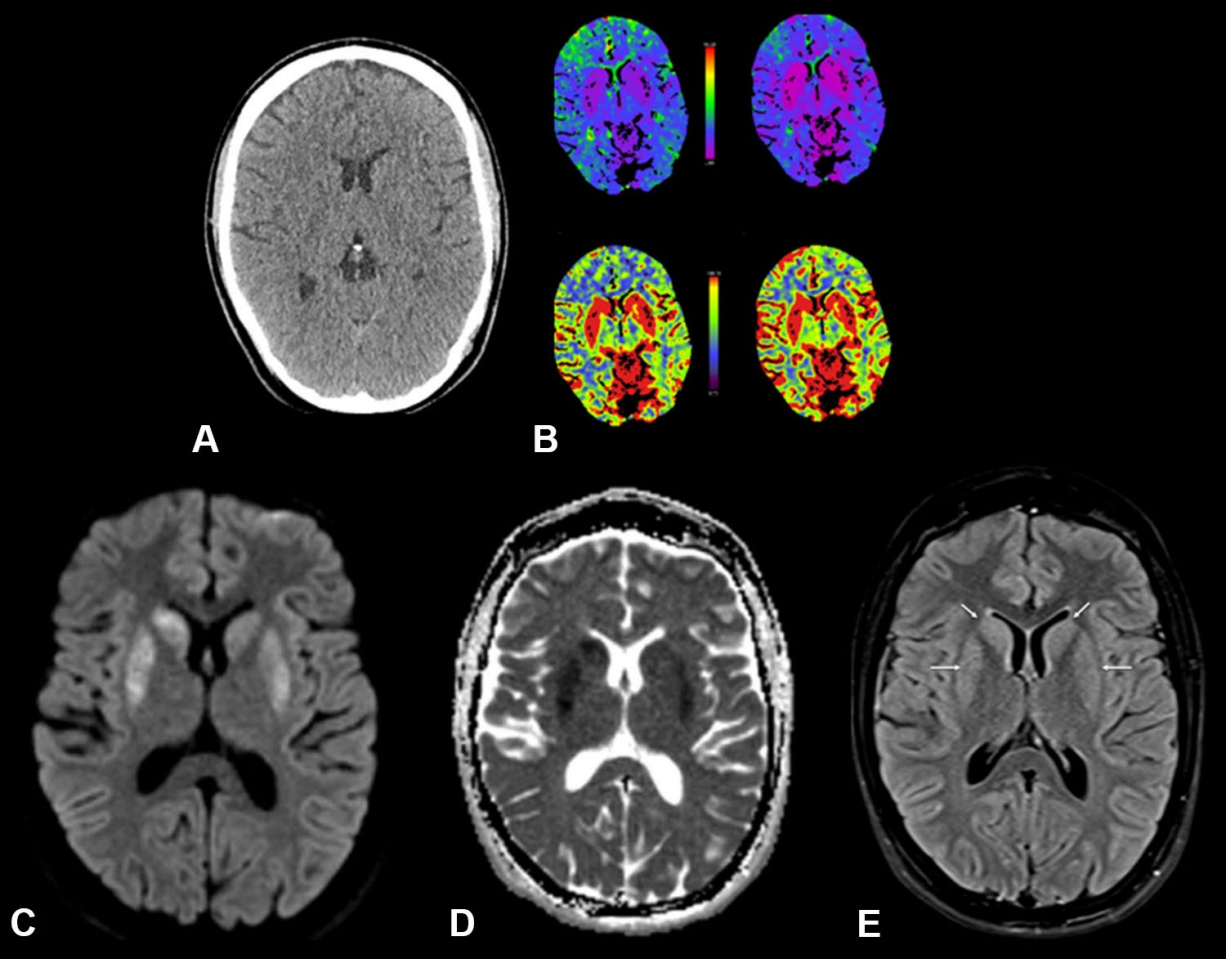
Axial NECT of the brain (ST 3 mm) with perfusion maps and MRI of the brain with DWI (ST 3 mm) and ADC maps and a FLAIR-weighted axial image (ST 4 mm). The axial native CT image of the brain shows diminished corticomedullary differentiation with involvement of the basal ganglia (A), confirmed in the CT perfusion maps (B). The MRI scan of the brain (28 h after the initial event) confirmed the severe hypoxic-ischemic encephalopathy with diffusion restriction of basal ganglia (arrows C and D) with demarcation on the FLAIR-weighted image (arrows in E)
